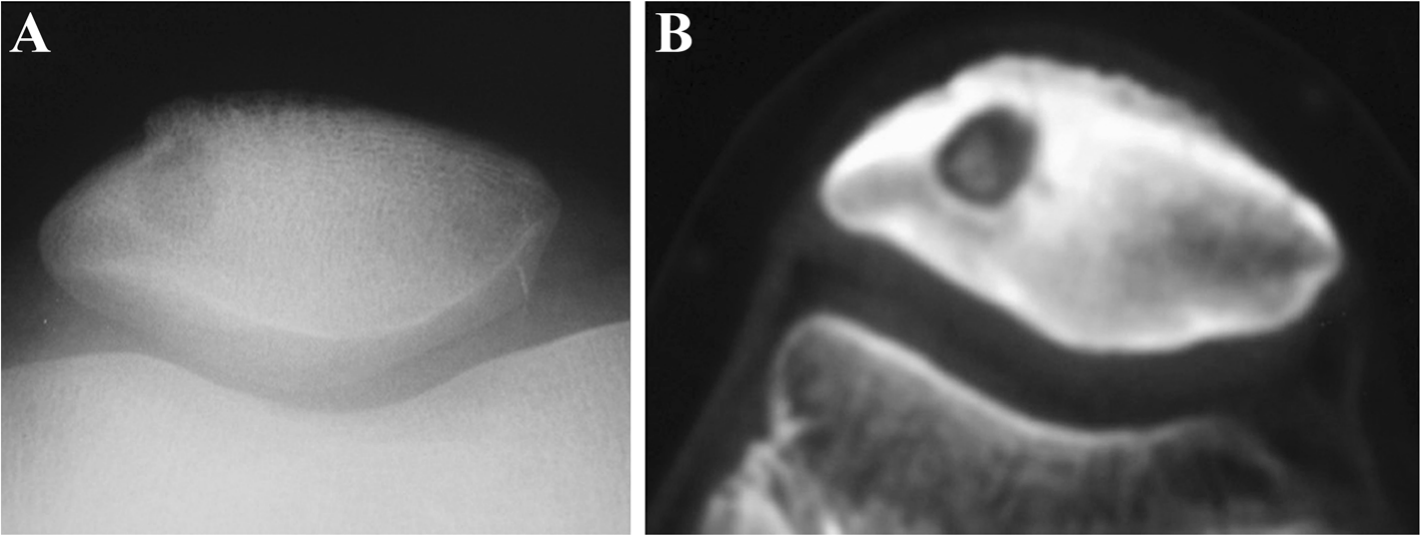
An intracortical osteoid osteoma of the patella. (A) On plain radiographs, a clear lesion with small fuzzy periosteal reaction is observed. (B) CT demonstrates the sclerosis surrounding the lesion.
